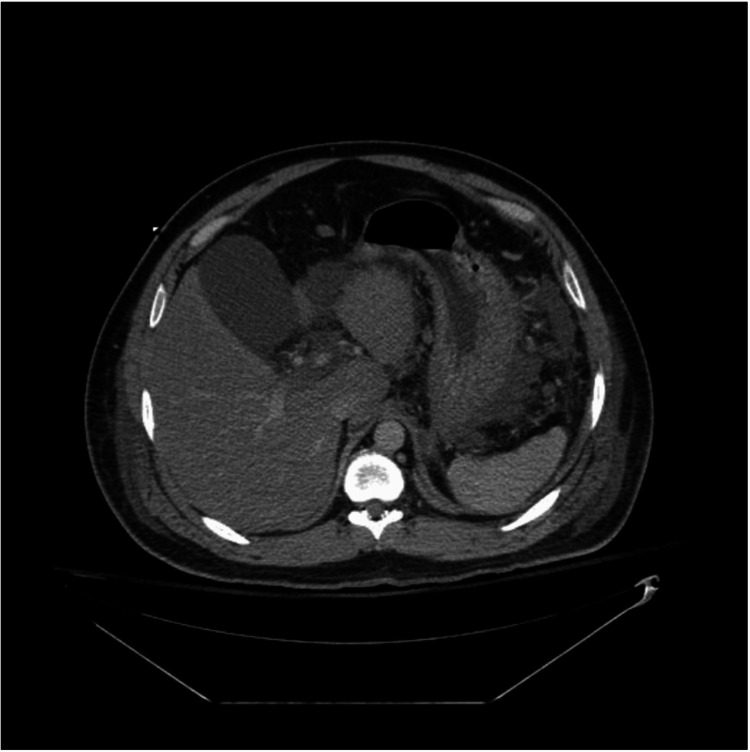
CT scan of the abdomen The CT scan showed interval progression of necrotizing pancreatitis within the pancreatic head, body, and tail with lobulated peripancreatic fluid collection, pancreatic parenchymal necrosis, possible focal hemorrhage within the pancreatic body and tail, and development of extensive peripancreatic, retroperitoneal edema.
